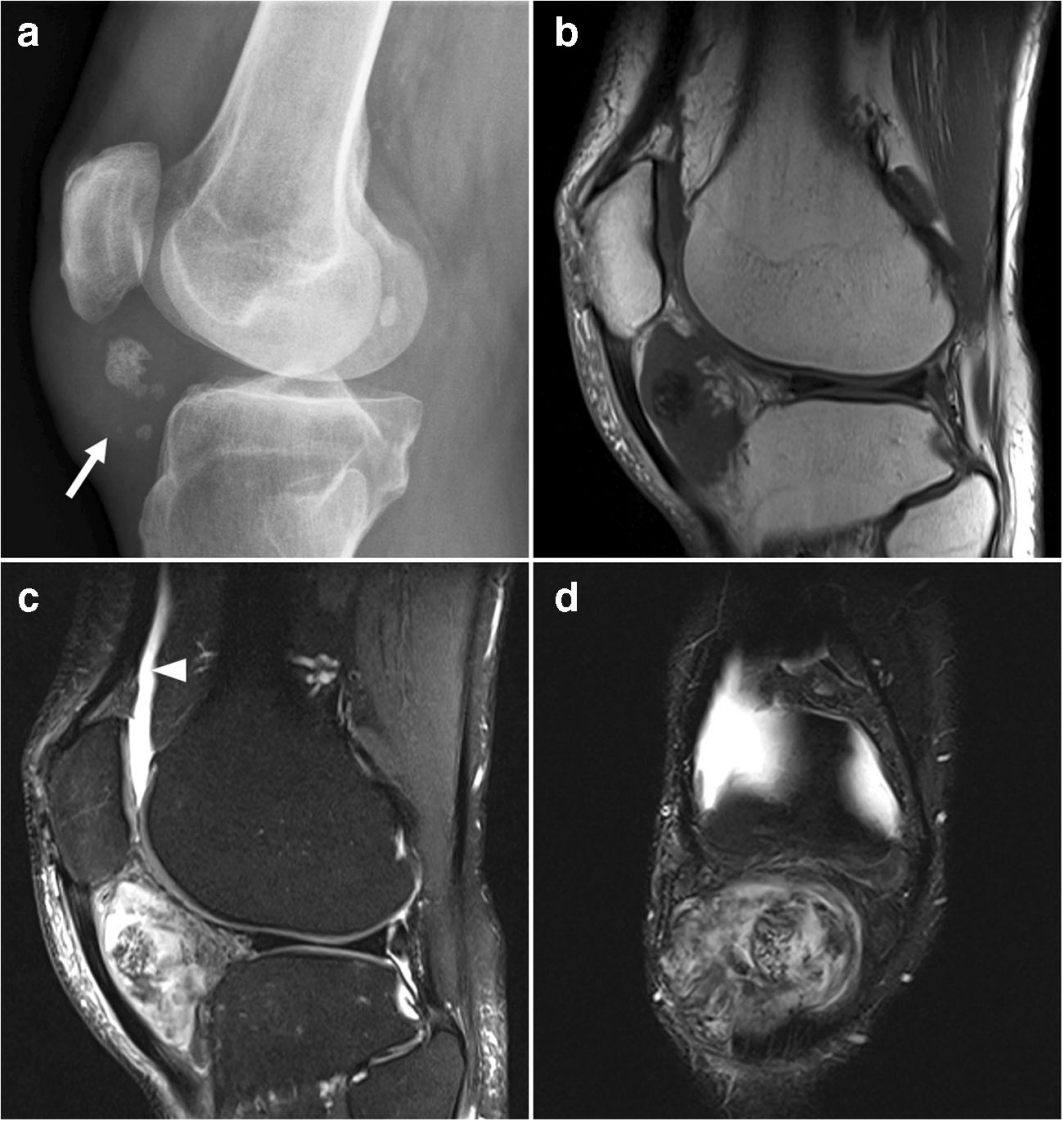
A 68-year-old man with Hoffa’s disease. a Radiography shows multiple ossified fragments (arrow) in Hoffa’s fat pad. b Sagittal T1-weighted image shows the largest ossified fragment in Hoffa’s fat pad, with surrounding low signal intensity. c, d Sagittal (a) and coronal (b) proton-density-weighted images with fat suppression show high signal intensity in Hoffa’s fat pad with areas of low signal intensity, corresponding to fibrin and hemosiderin
